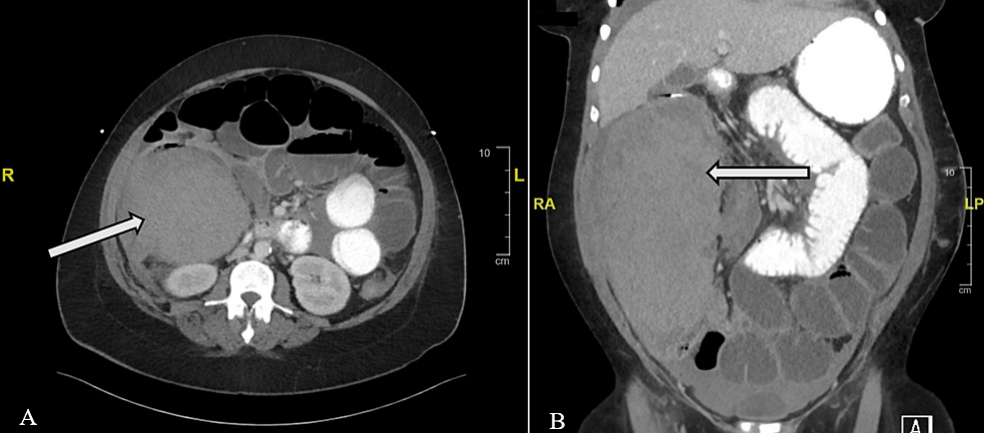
This figure shows coronal (A) and sagittal (B) CT scans with iopamidol contrast showing a massive colonic hematoma (see arrows). Subtle active bleeding is noted within the hematoma. It appears to be pushing into the dilated right hemicolon. There is also a moderate amount of free fluid in the abdomen and pelvis.
